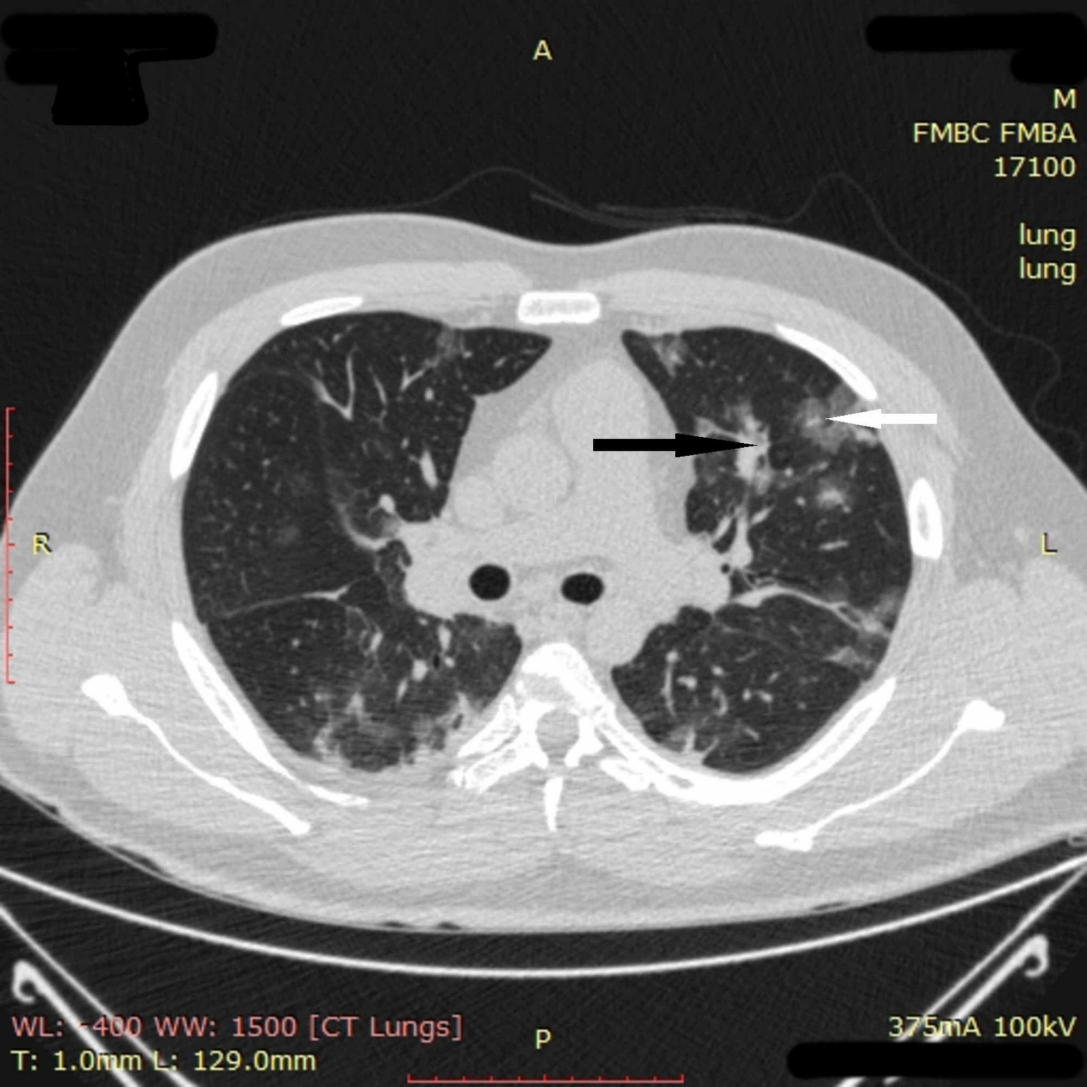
Chest CT imaging of patient 3 on admission Chest CT on admission revealed multiple ground-glass opacities (white arrow) and areas of pulmonary consolidation (black arrow) in all segments of the lungs CT: computed tomography
